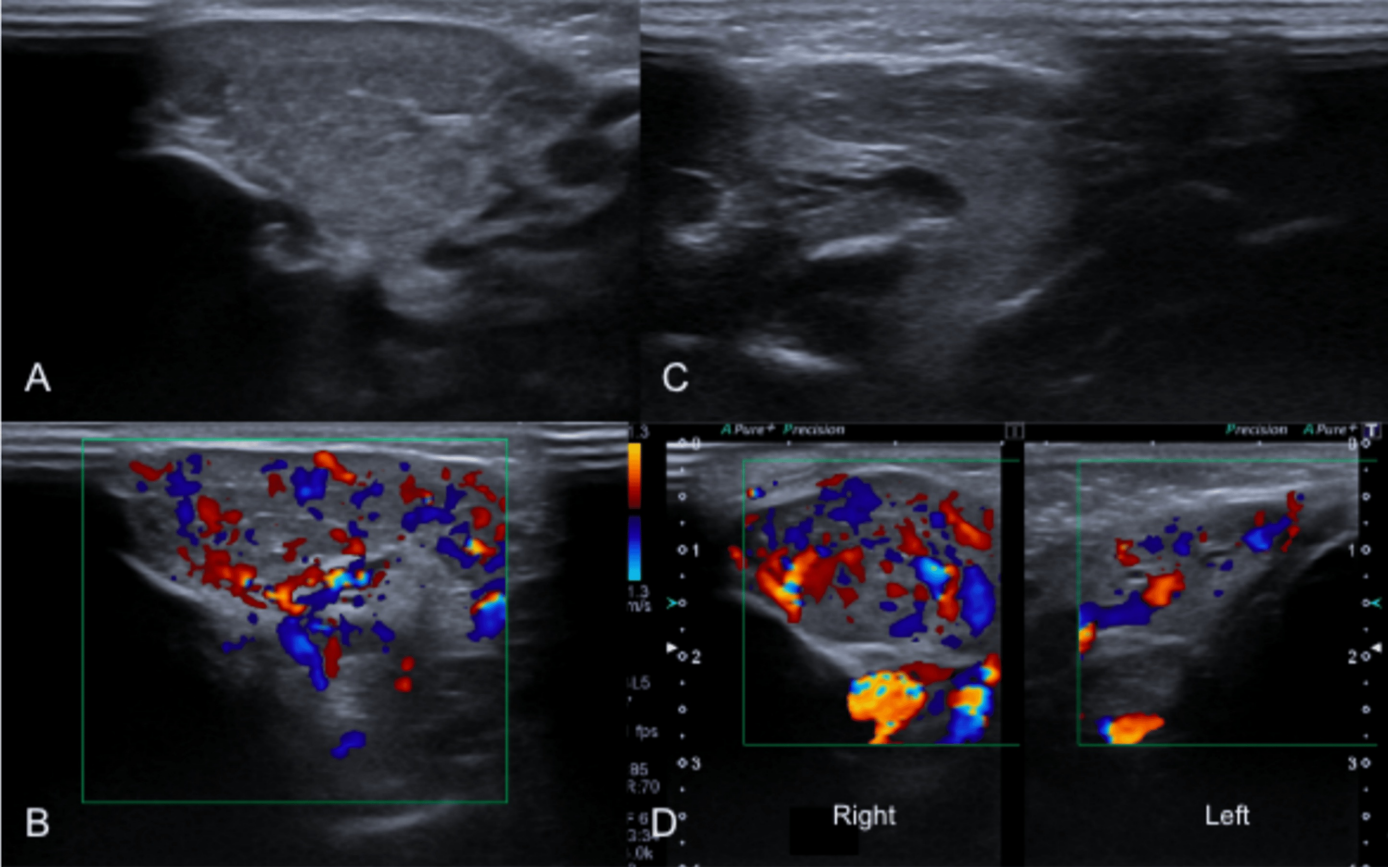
Soft tissue ultrasound of face. (A) The right parotid gland is diffusely enlarged and hypoechoic. (B) The color Doppler shows prominent internal vascularity. (C) Left parotid gland was normal. (D) In color Doppler there is a comparative increase of vascular flow in the right parotid gland compared to the left.
